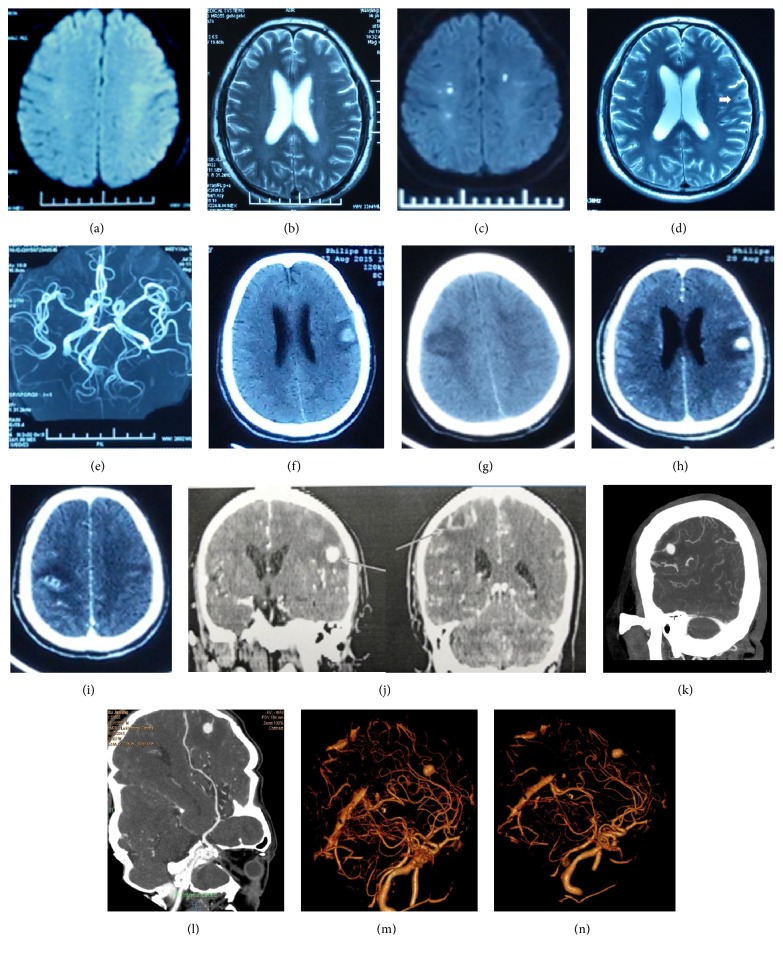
Dynamic changes on brain MRI and CT imaging. ((a) and (b)) On admission, DWI or T2 sequences of MRI showed hyperintense signal intensity in the right centrum semiovale and hyperintense lacunar lesions adjacent to the left lateral ventricle. ((c) and (d)) On day 7 of admission, MRI showed hyperintense signal intensity in the left cerebellar hemisphere, left temporal lobe, and bilateral frontal-parietal lobe. A flow void was found in the left hemisphere (arrow). (e) MRA did not show any abnormality in the intracranial arteries. ((f) and (g)) On day 11 of admission, CT scan revealed high density nodules in the right frontal-parietal region and a low density shadow in the right parietal lobe. ((h)–(j)) On day 20 of admission, Contrast-enhanced CT showed high density nodules surrounded by edema in the left frontal-parietal region and a ring-enhancing nodule in the right parietal lobe with peripheral edema (arrow). ((k)–(n)); CT angiography showed an aneurysm on a cortical branch of the left middle cerebral artery.
